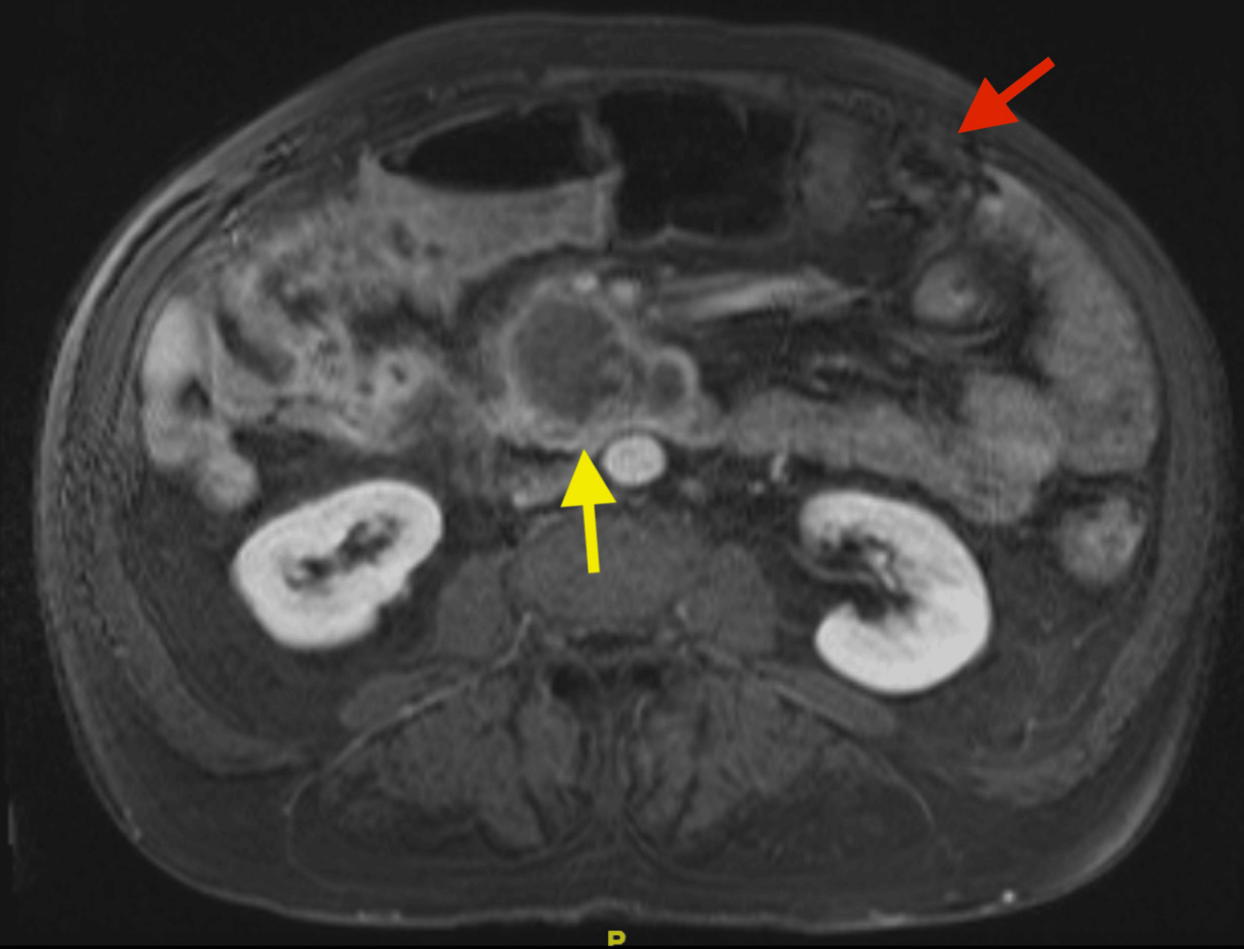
Magnetic Resonance Imaging of the Abdomen and Pelvis With Contrast Yellow arrow: Pancreatic head mass; Red arrow: Transverse colon mass at the prior partial colectomy anastomosis site.
